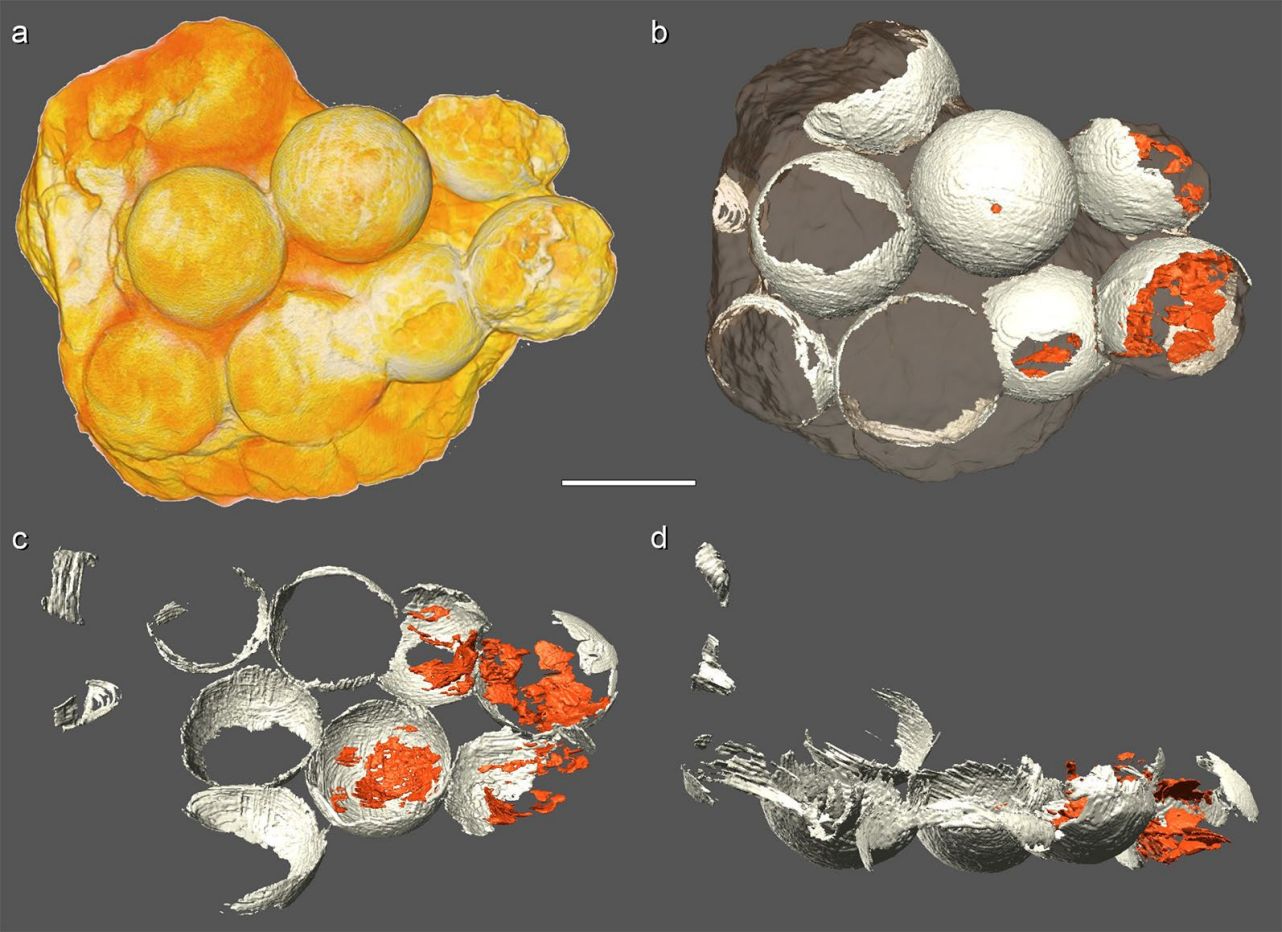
CT scans of the best-preserved egg-clutch (CPPLIP 1798). (a) 3D rendering of the bottom of the clutch in CPPLIP 1798. (b) General view of the clutch at the same orientation, showing the external part of the lower hemisphere of the eggs. (c) Top view of the lower egg row, showing the inner part of the lower hemisphere of the eggs. (d) Lateral view of CPPLIP 1798, showing lower egg row with higher shells at both ends and part of the upper egg row. In dark orange, eggshell fragments within the eggs. Note that the incompleteness (“holes”) of some eggs in B and C are due to the lack of the eggshells and/or poor resolution of the CT scan. In A the shape of each egg is maintained due to the sedimentary internal mold. Scale bar 10 cm.
